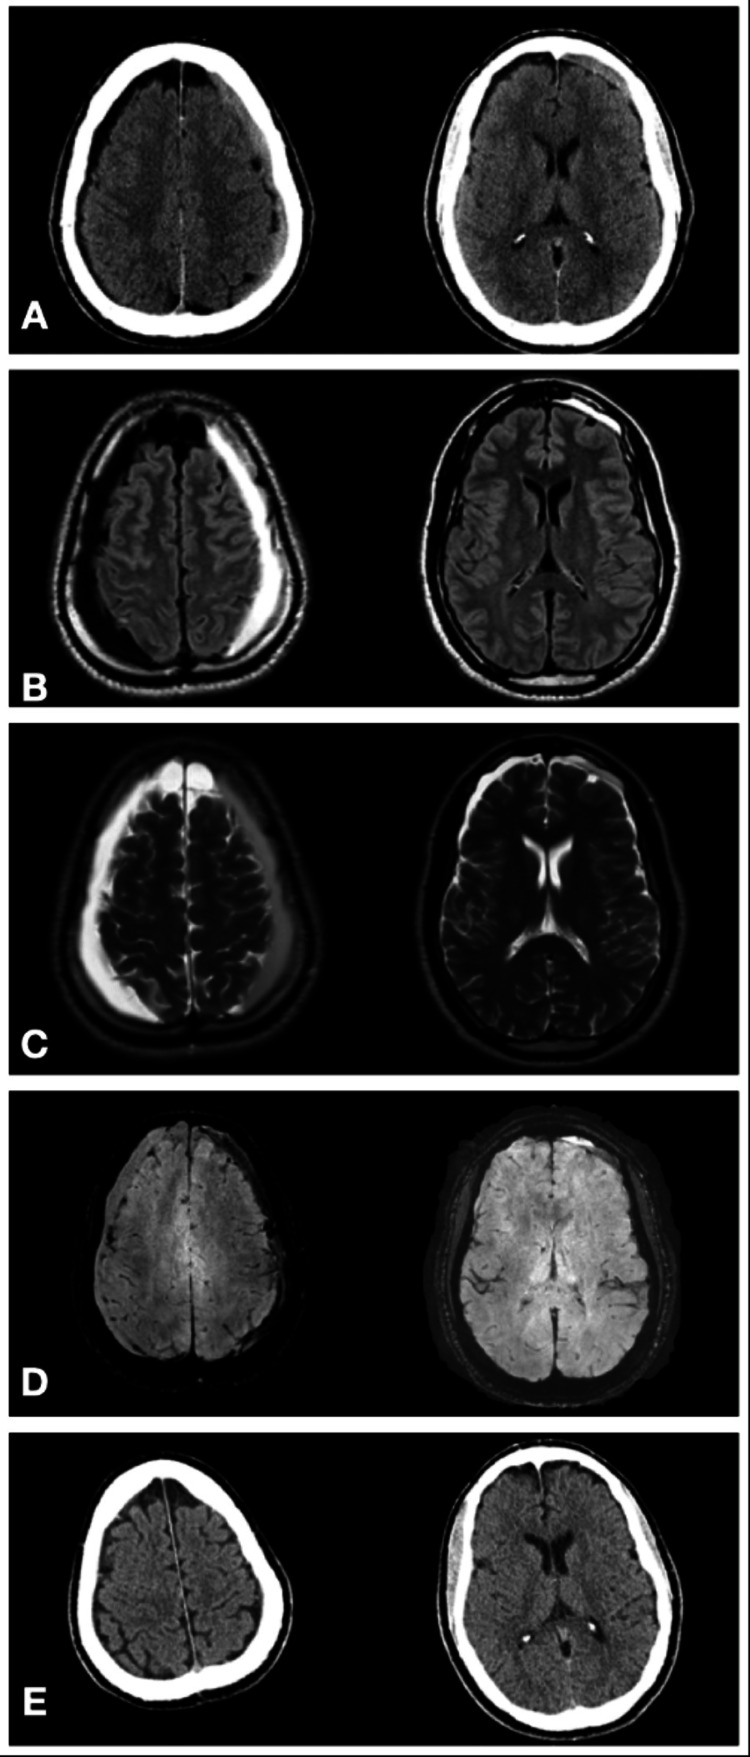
Panel A: CT at admission showing bilateral subdural hematomas, Panel B: T1 MRI at admission, Panel C: T2 MRI at admission, Panel D: SWI MRI at admission, and Panel E: CT at three-month follow-up after middle meningeal artery embolization showing resolution of bilateral subdural hematomas SWI: susceptibility-weighted imaging
